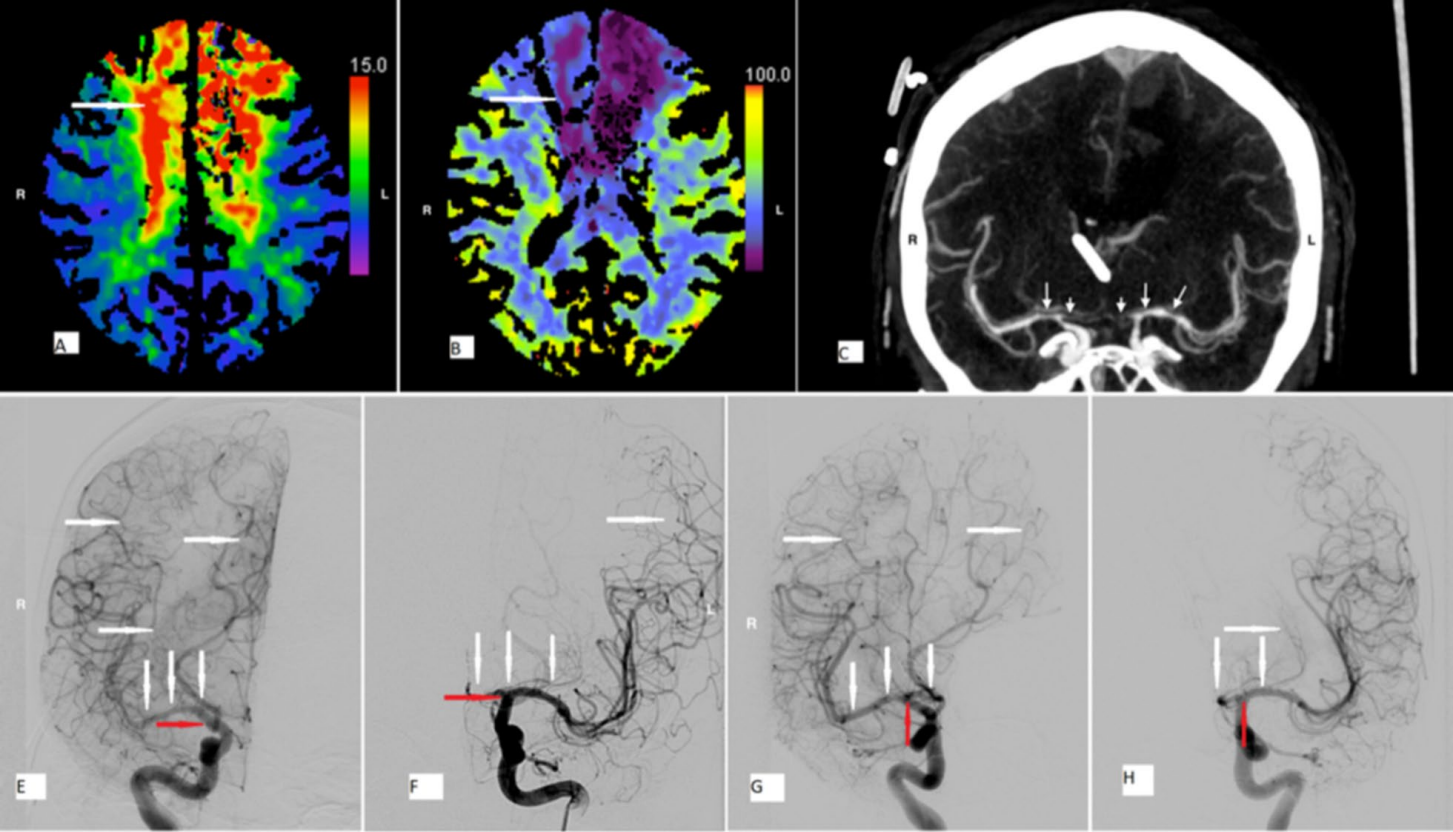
Sodium nitroprusside effect on cerebral perfusion and vasospasm in a patient after severe subarachnoid hemorrhage. Upper panel—CT perfusion a regional mean transit time and b cerebral blood flow during treatment interruption revealed high-grade perfusion disorder and brain at risk in the anterior cerebral artery territory (arrows). A brain infarction in the left anterior cerebral artery territory existed since clipping. c CT angiogram during molsidomine interruption confirmed transcranial Doppler sonography findings of the high-grade vasospasm in the anterior and the middle cerebral arteries. Arrows indicate vasospasm in both arteries. Lower panel—e–h Digital subtraction angiography after restarting molsidomine (1.5 h later; transcranial Doppler sonography was normal showing the anterior cerebral artery segment with no remaining perfusion gap and well filled the internal carotid and the middle cerebral artery. Left-sided aneurysm clipping induced truncation of the A2 segment of the anterior cerebral artery without perfusion disorder in the remaining territories. Arrows point at the full reversal of the high-grade spasm of the internal carotid artery at the bifurcation to the middle cerebral artery bilaterally and the anterior cerebral artery (right)
